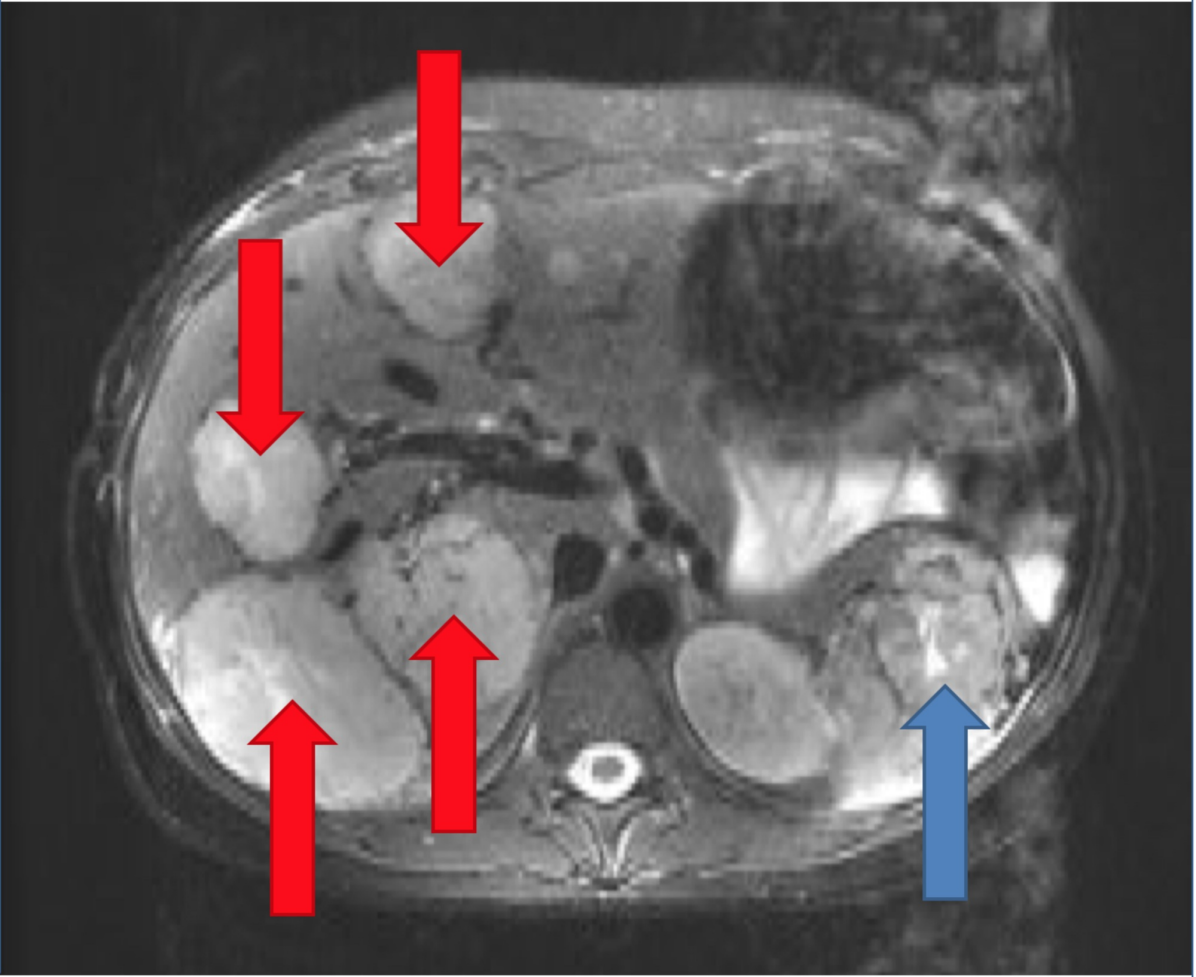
Magnetic Resonance Imaging of the Abdomen Significant for a large, predominantly solid, pancreatic tail lesion with central necrosis (blue arrow). Large liver lesions are present in the majority of the segments of the liver suggesting neuroendocrine metastases (red arrows).
